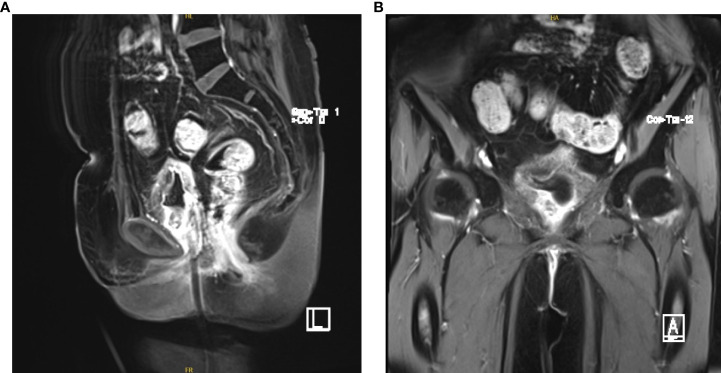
(A, B) Pelvic magnetic resonance imaging (May 17, 2022).
